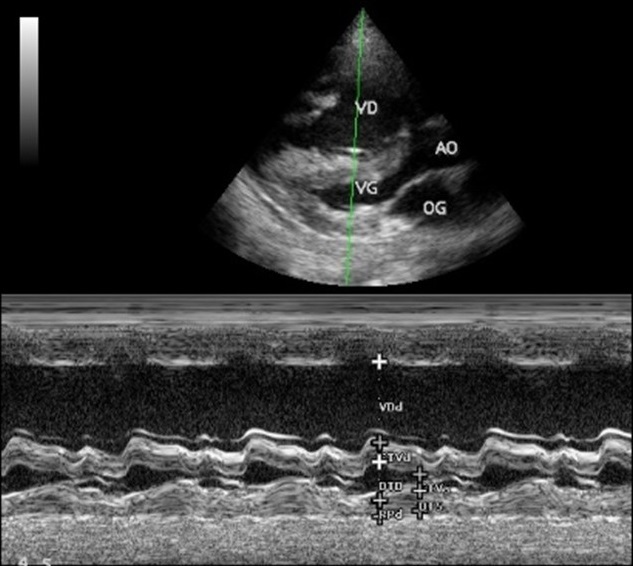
Pré-opératoire: coupe parasternale grand axe, mode bidimensionnel (image en haut) et mode TM (image en bas); Ventricule droit (VD) dilaté avec paroi antérieure épaissie; Ventricule gauche (VG) apparaît relativement petit avec une bonne fonction systolique; AO: aorte; OG: oreillette gauche
